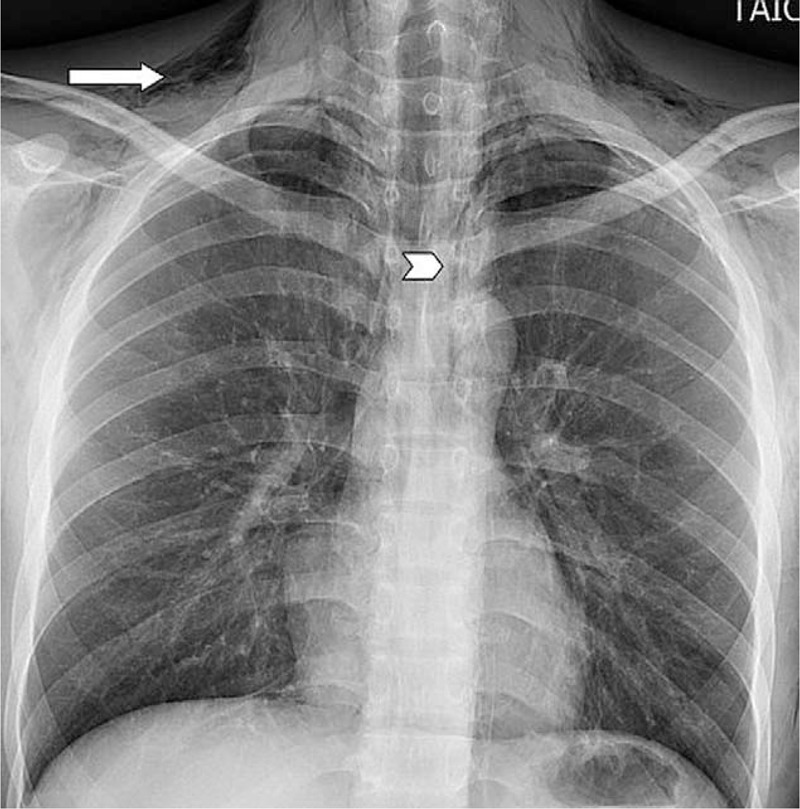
Chest x-ray revealing diffuse subcutaneous emphysema in the soft-tissue layers of the neck and upper thorax (arrow) and the linear distribution of air along the mediastinum (arrowhead).
